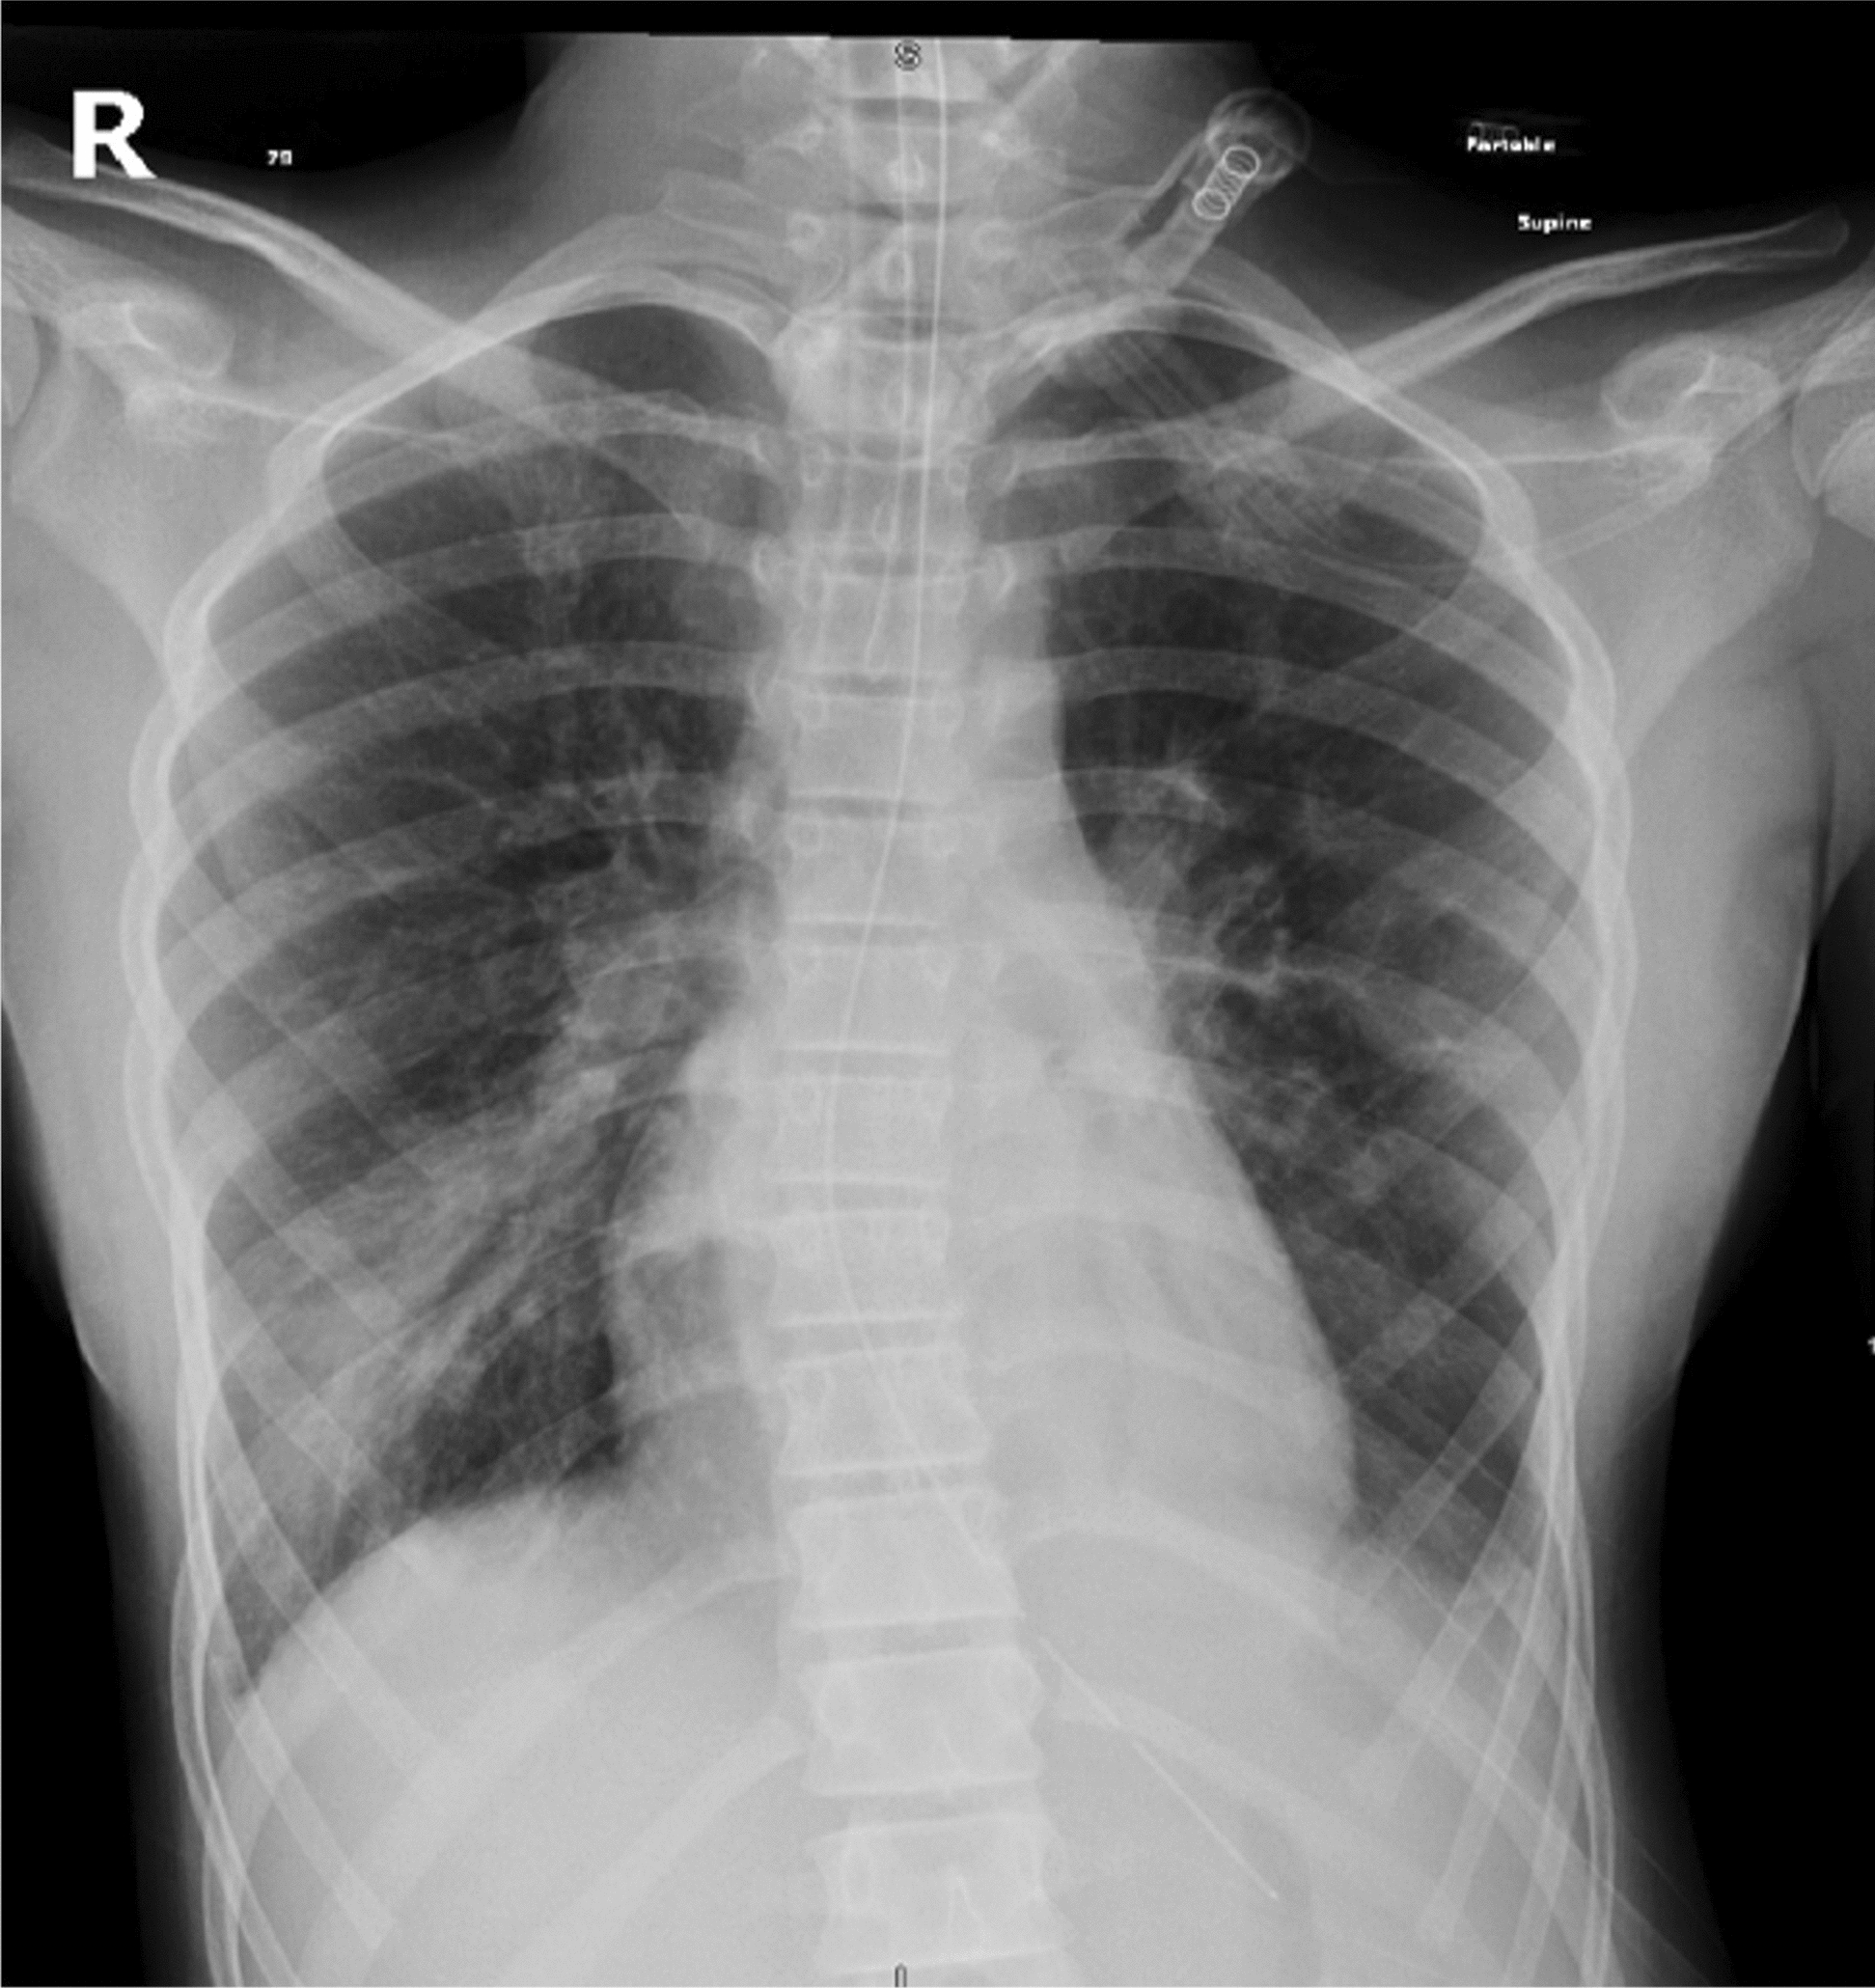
Chest x-ray showing bilateral hyperinflation of the lung and right middle lobe infiltration
